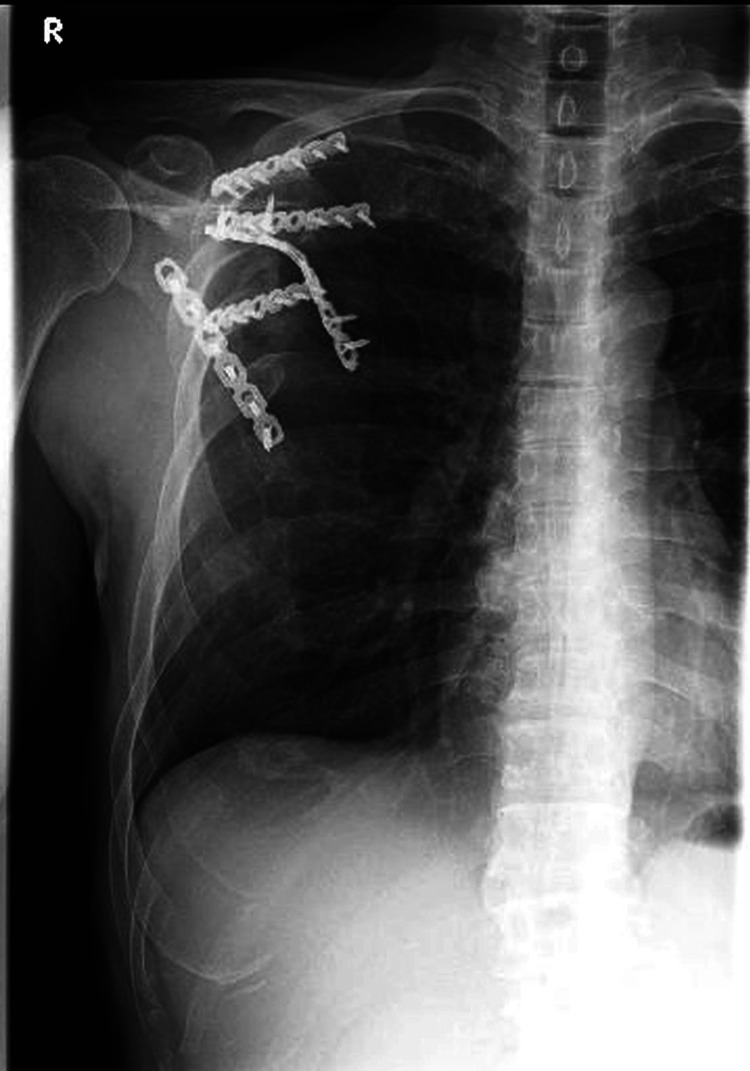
Chest and scapular X-rays at 1.5 years postoperatively The chest X-ray shows no abnormalities, and the rib fractures have achieved bone union. The scapular fracture has also healed, with no abnormalities observed in the implants
